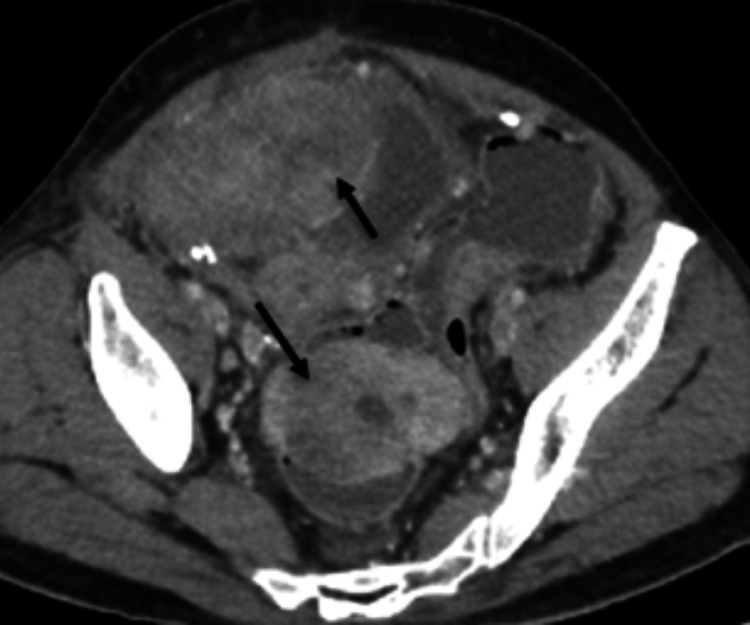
Axial CECT image in venous phase showing lobulated heterogeneously enhancing masses in the mesentery and pelvis (black arrows) CECT: contrast-enhanced computed tomography
